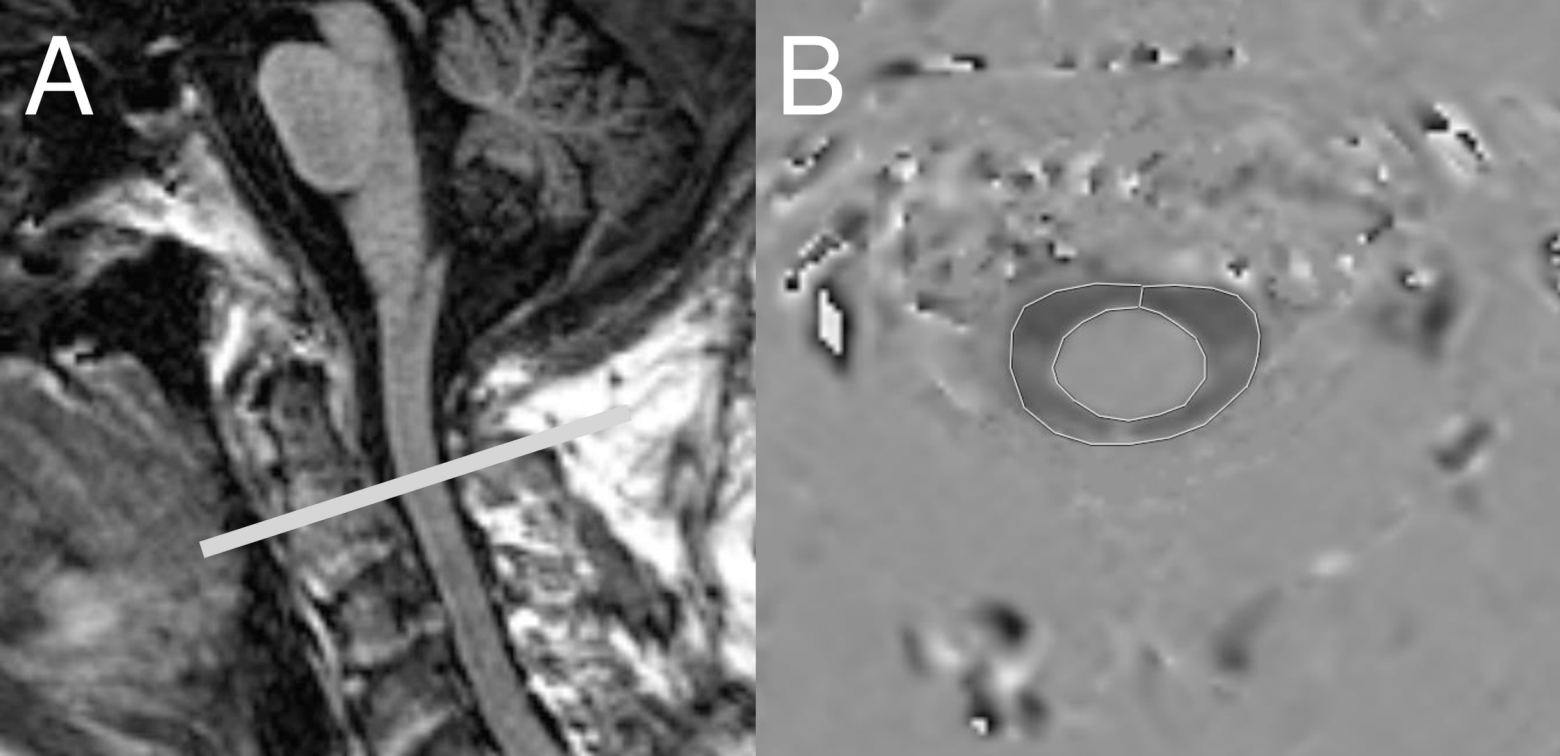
(A) Level of PC-MRI acquisitions. PC-MRI was performed perpendicular to the cervical spinal canal at level C2, which is typically the closest level to the intracranial compartment where the assumption of the spinal canal as a rigid, cylindrical tube can be made when estimating MRI-dP. (B) ROI outlining. The subarachnoid space was manually defined from phase images with a region of interest (ROI).
